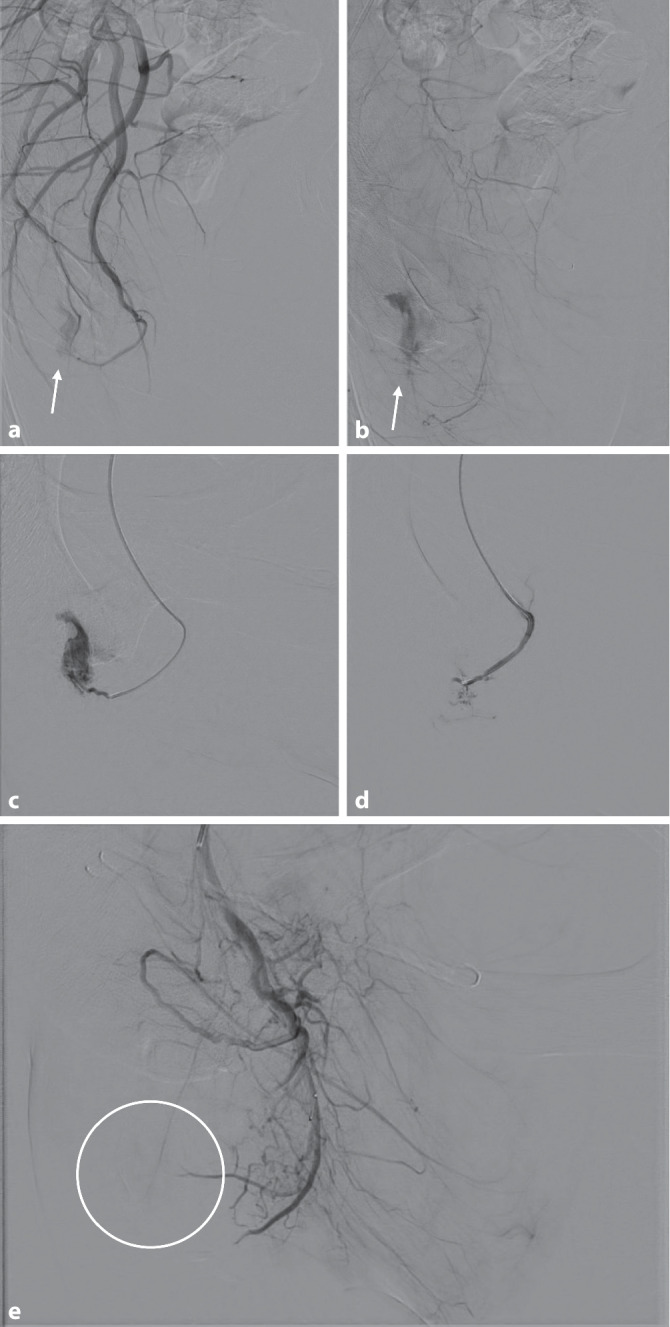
Nach Entfernung des Kompressionsverbandes am ersten postinterventionellen Tag zeigte sich die Shunthöhle sonographisch deutlich regredient. Bei klinisch stabilem, asymptomatischem Zustand erfolgte die Entlassung des Patienten nach Hause.
